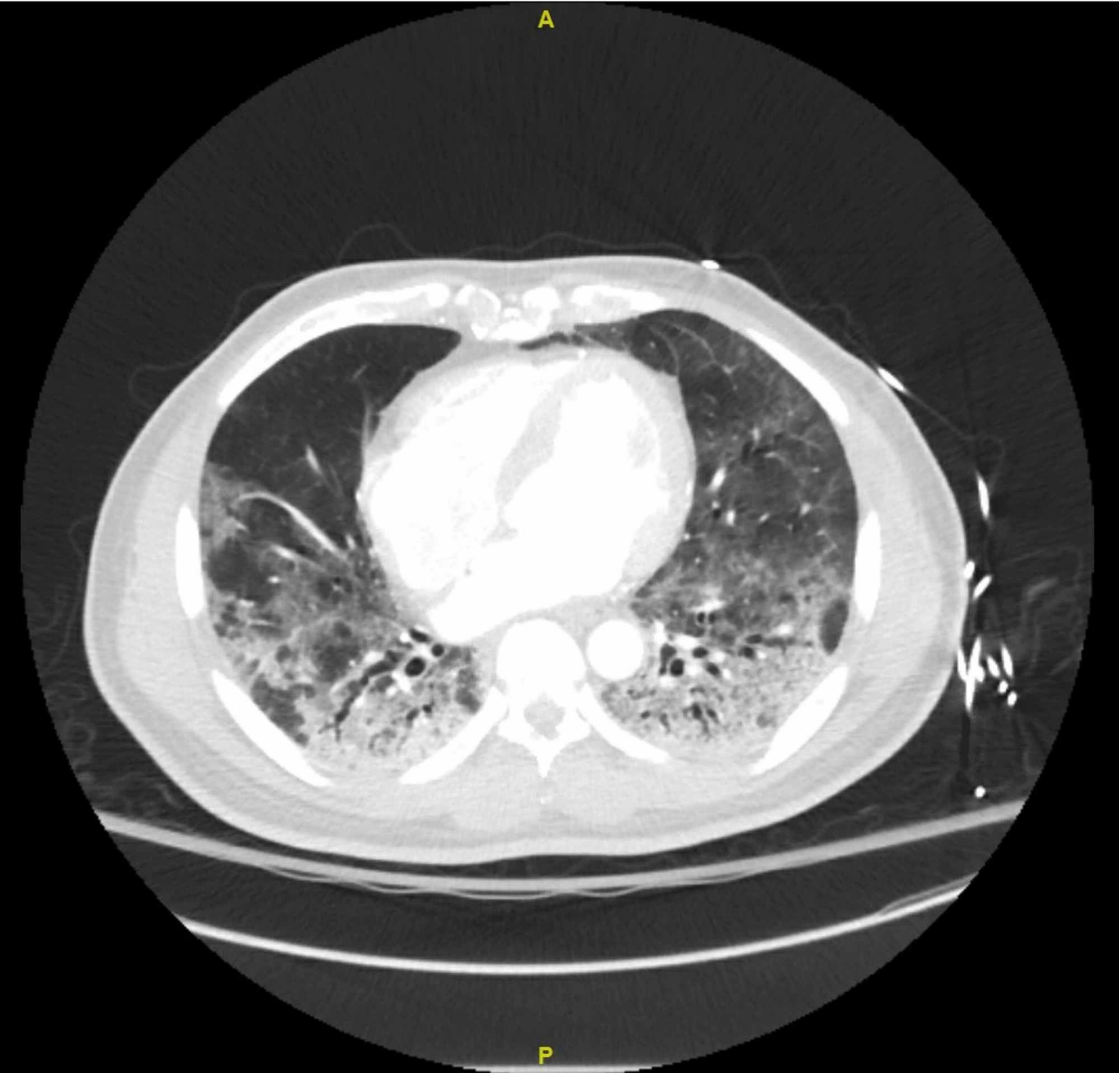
CT scan of the chest with intravenous (IV) contrast, transverse view Demonstrating diffuse ground-glass opacities in bilateral lung fields, most extensive in the bilateral lower lobes with mixed attenuation consolidations and accompanying traction bronchiectasis.
